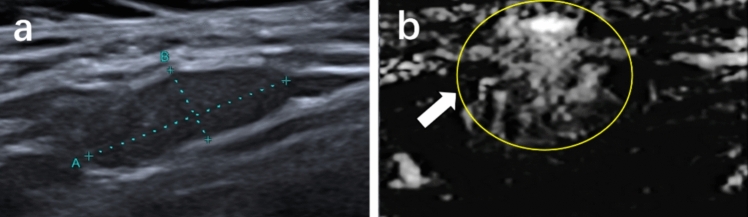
Metastatic lymph node from a 48-year-old woman. a Conventional ultrasound. b Superb microvascular imaging (SMI), the lymph node exhibits misty vessel distribution
